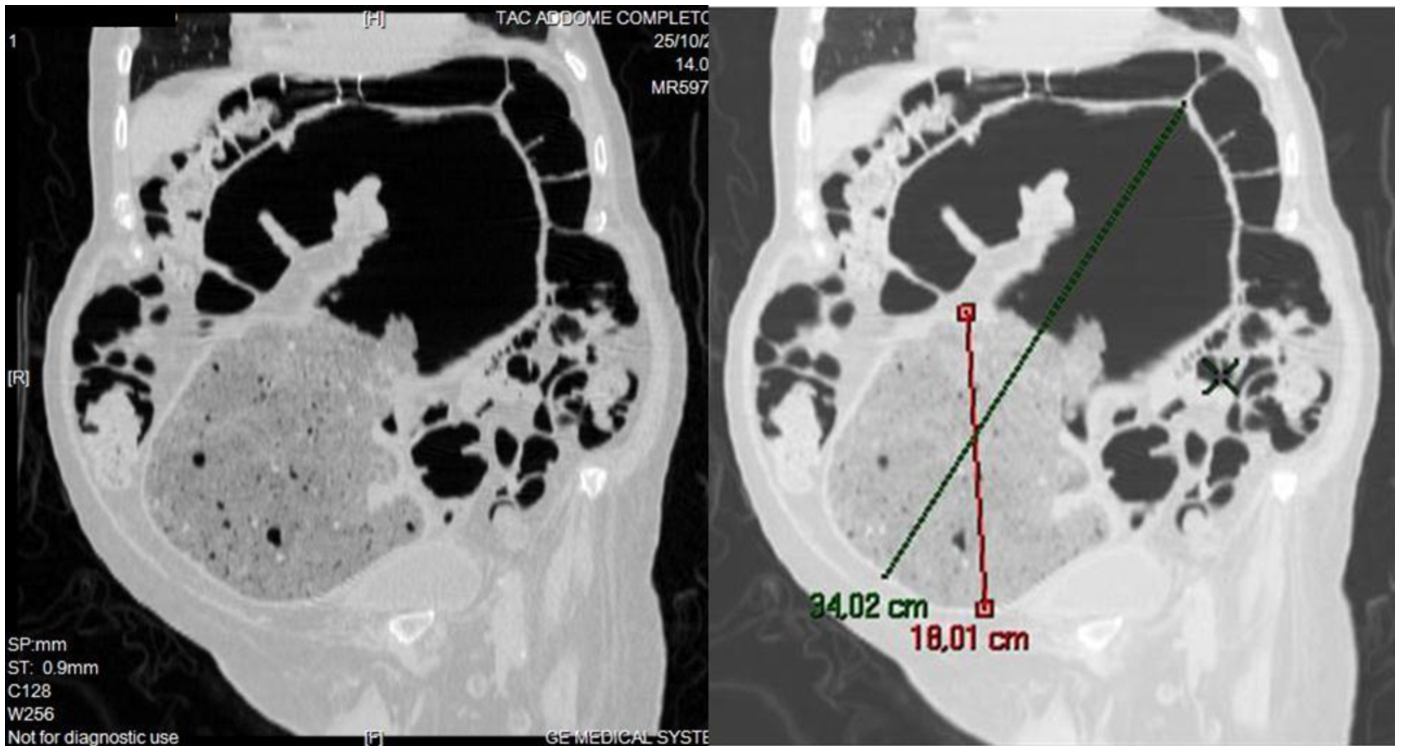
Abdominal CT scan with multiplanar reconstruction showing the persistence of a large faecal impaction, over 18 cm in extent, located in the sigmoid colon, as well as a massive dilatation of the colonic wall and the descending sigmoid colon forming a twisting loop.
